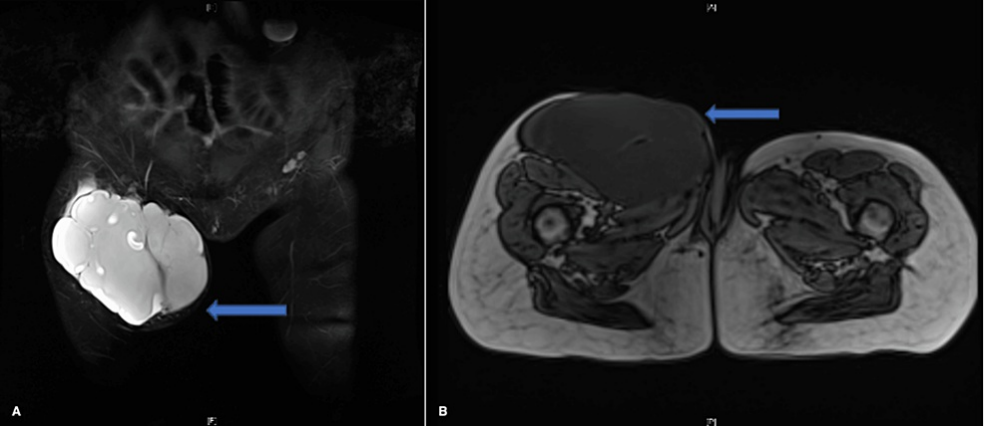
MRI of right thigh lesion A: Coronal view of thigh lesion. Well-defined multi-septated mass measuring 14 x 7.6 x 11.4 cm, deep to the skin, and separate from deep fascia and anterior medial right thigh with compression of the anterior compartment muscles. Heterogenous marrow pattern is demonstrated with enhancement of uncertain nature. B: Axial view of thigh lesion
